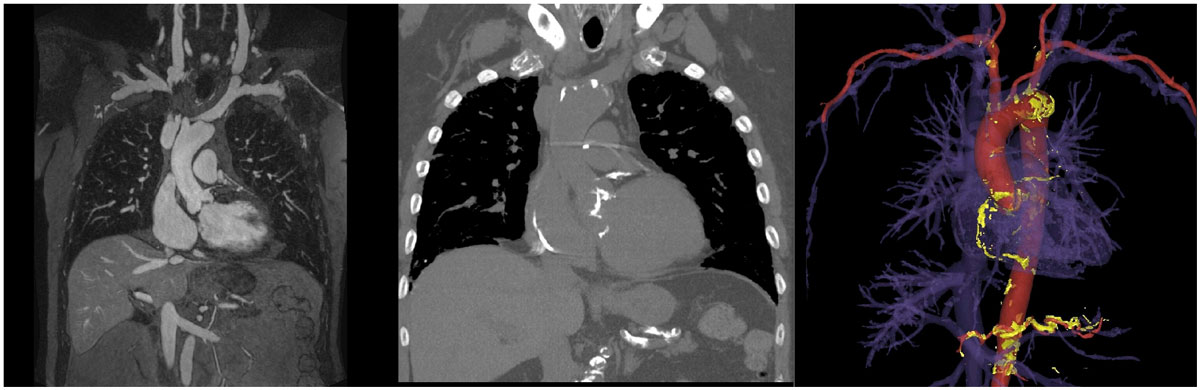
FEMRA (left), non-contrast CT (middle) and Volume rendered FEMRA with calcium overlay (right) in an 84 year old male patient with calcific aortic stenosis who had successful TAVR placement.
